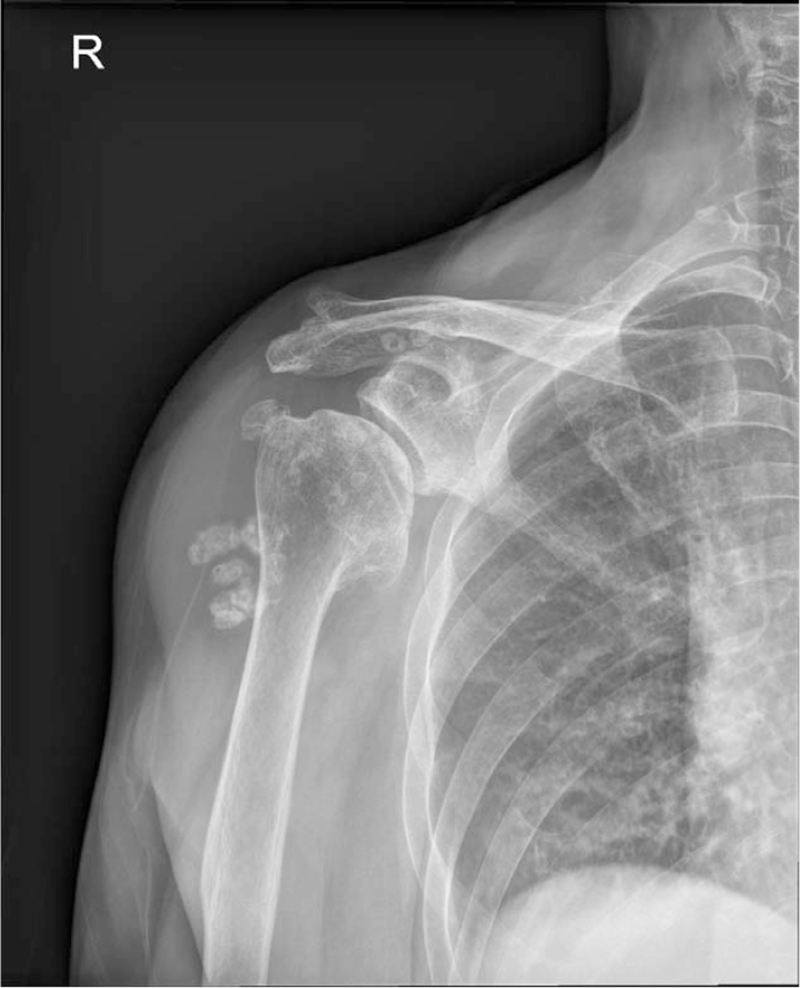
Initial shoulder radiograph shows joint space narrowing and subchondral sclerosis of the glenohumeral joint. Loose bodies were located in the subdeltoid bursa and subacromial bursal area.
